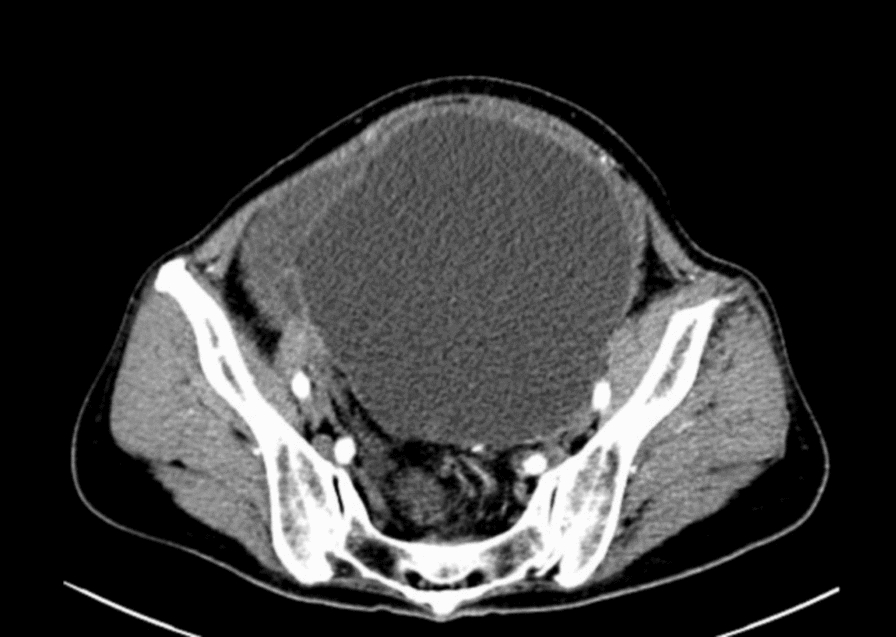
Preoperative axial computed tomography scan of the pelvis showing a large cystic mass and associated ascites
